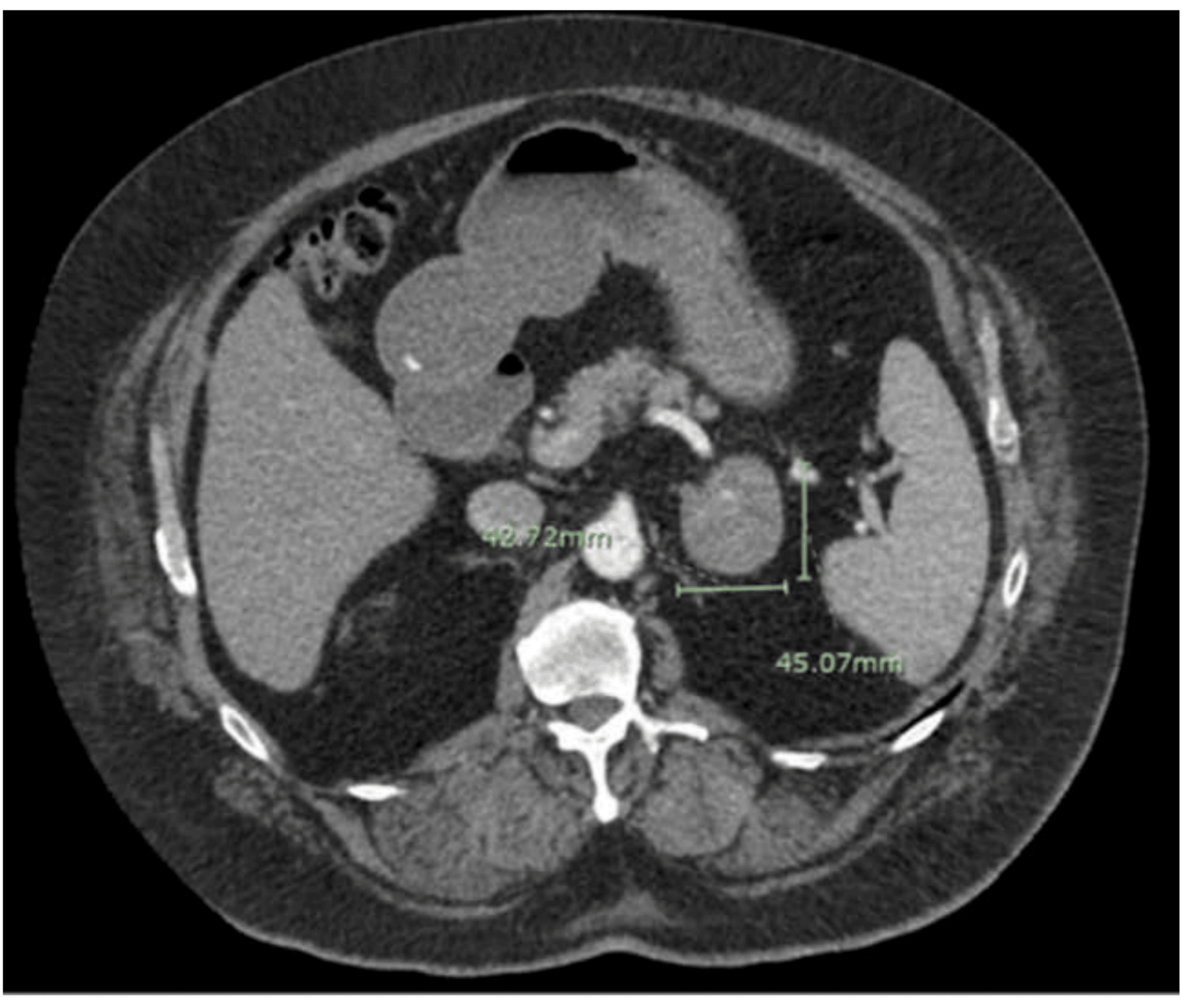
Abdominal CT 3-phase demonstrating a 4.2 x 4.5 cm adrenal mass.
